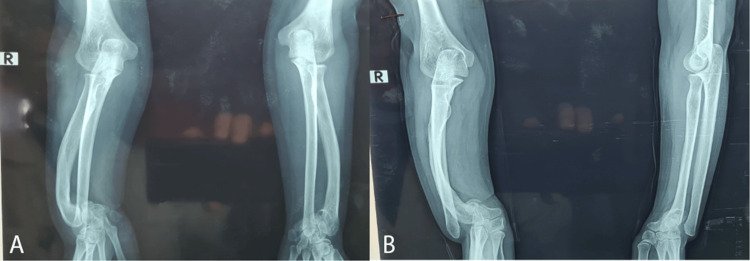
Radiographic images of both upper extremities: (A) anterior view and (B) lateral view
